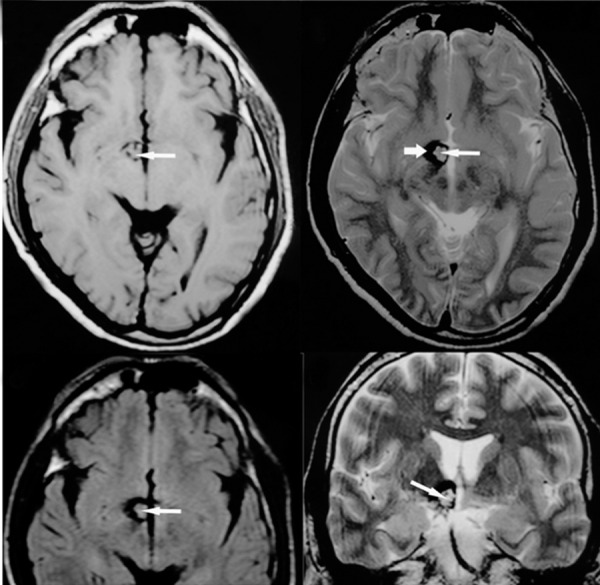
A, Axial T1 weighted; B, Axial T2 weighted; C, Axial FLAIR; and D, Coronal T2 weighted images show a small well-defined mass on the right side of the hypothalamus with a mixed intensity core appearing like “popcorn” (long arrow) surrounded by a low signal intensity hemosiderin rim (short arrow) seen on T2 weighted images with no edema or mass effect as noted on FLAIR images.
